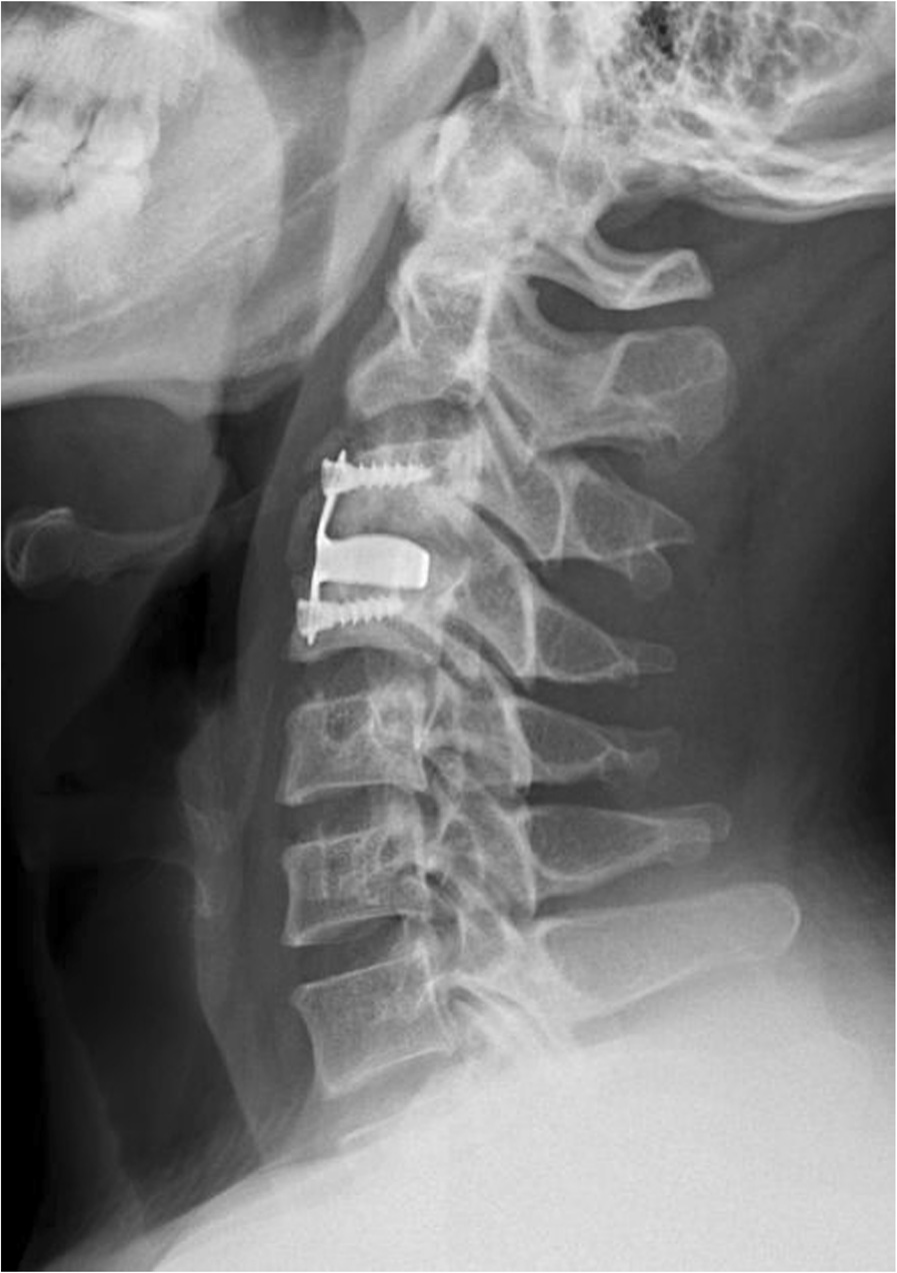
Lateral radiograph showing C3 and C4 screw loosening and heterotopic ossification formed 5 months after the operation
